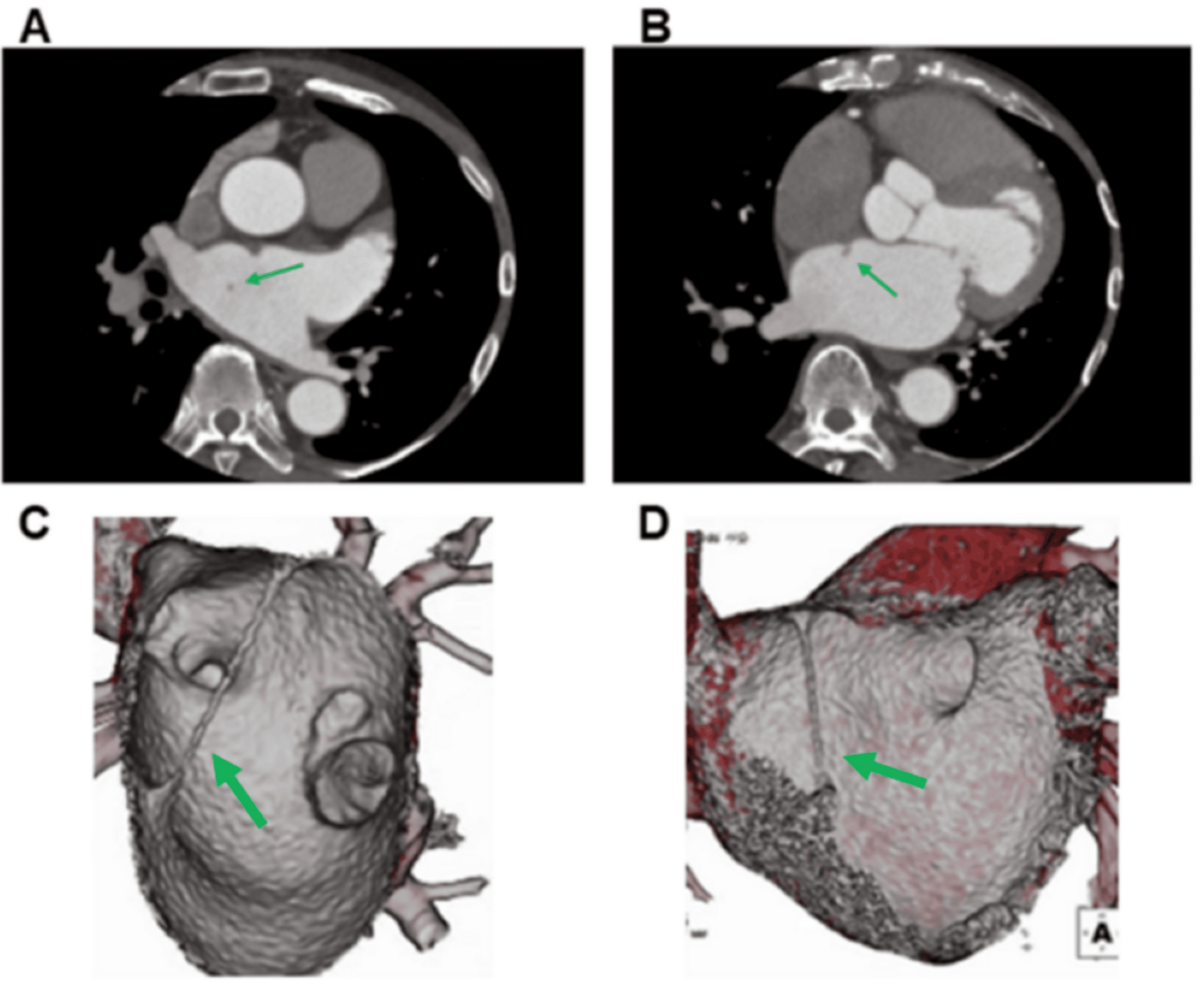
A cord-like structure connecting the fossa ovalis to the left atrial roof was observed on contrast-enhanced CT (A, B) and reconstructed 3D CT image of the inner (C) and anterior view (D). The green arrow indicates the atrial band.
